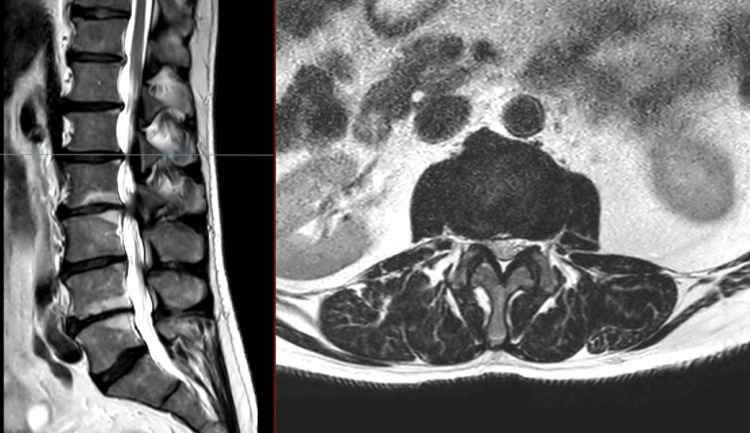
T2 MRI of the lumbar spine: sagittal (left), axial (right) at L1/2 No evidence of spinal stenosis, no compression of cauda equina were noted.
